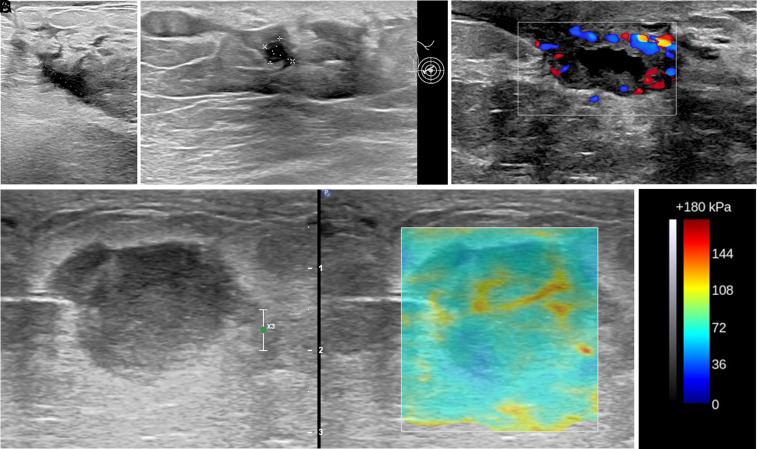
Targeted ultrasound images from (A) through (D) depicting different grades of idiopathic granulomatous mastitis (IGM) on different patients. (A) A fistula tract shows the drainage of purulent content on the background B-mode image and color Doppler map displays ongoing hyperemia within the wall of the tract. This finding corresponds to Grade II. (B) Hypoechoic solid-like mass measuring about 3.4 cm on long axis with diffuse increment on echogenicity of surrounding tissue obtained at gray-scale ultrasound at 9 o’clock position of the right breast in a 28-year-old postpartum woman presenting with denial of breastfeeding by her baby on the affected breast. Following 3 weeks of conservative treatment due to a lack of symptom resolution with progression in the size of the lesion, a biopsy was performed, and the result was IGM. This lesion demonstrates the earliest course of disease initiation and corresponds to grade I. (C) In another patient with a history of periductal mastitis on the contralateral breast prior 2.5 years was referred to the tertiary university hospital for further work-up of the lesion on the right side. The abscess formation (measurement markers) is clearly depicted within the extremely IGM-involved periareolar breast side. These findings correspond to grade III. (D) Elastosonographic image of the biopsy-proven IGM lesion. Color box (E) indicates the stiffness of tissue based on elasticity properties of the region of interest with red depicting the hardest and blue the softest.
